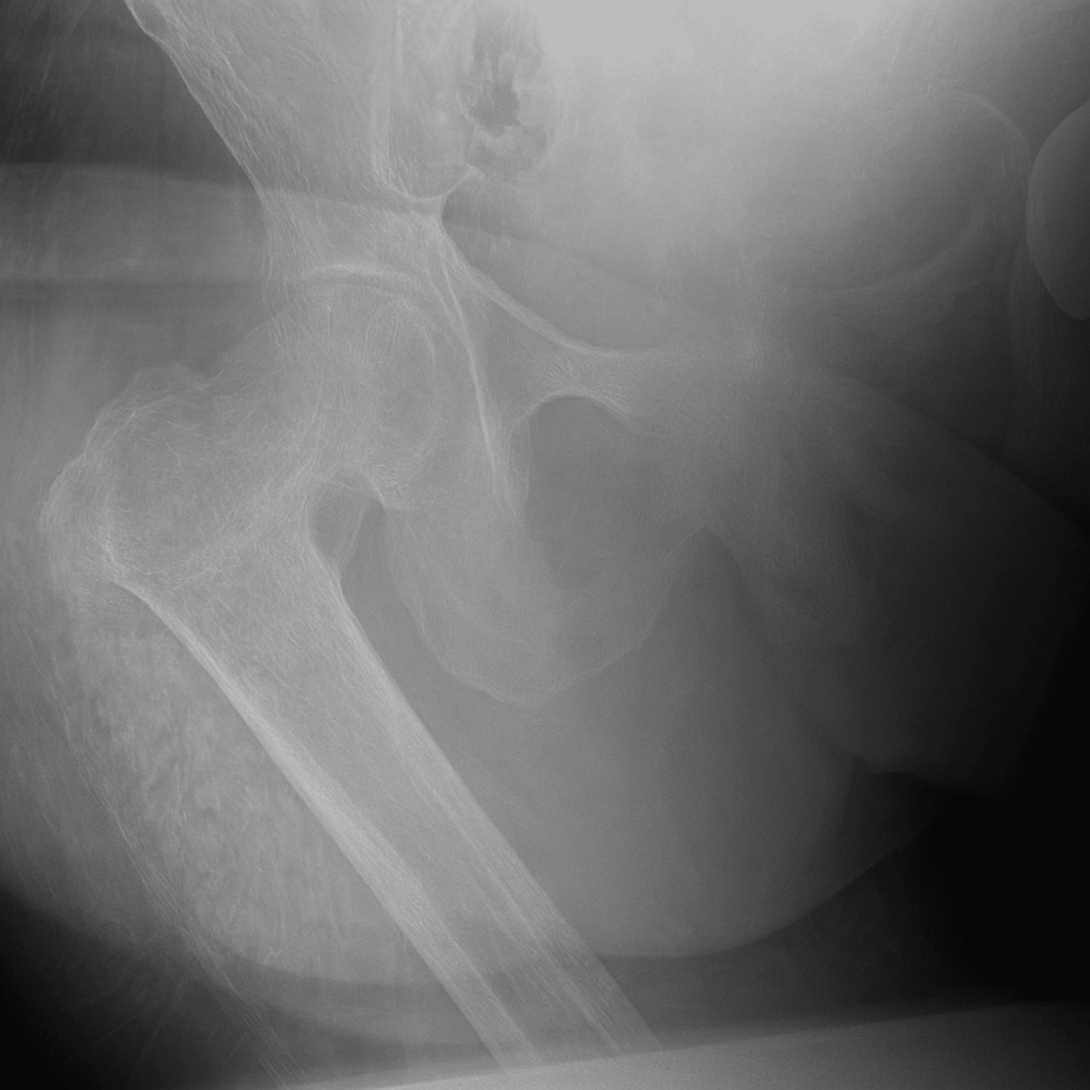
Preoperative radiograph showing a comminuted intertrochanteric fracture
